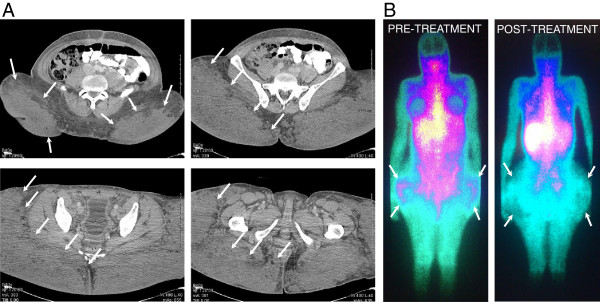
Imaging data showing extensive grannulomatous disease in the hip region. A - Axial computed tomography (CT) images showing large amounts of diffusely infiltrating granulomatous tissue (arrows). B - Gallium-67 uptake scan showing diffuse tracer uptake in the perigluteal soft tissue, corresponding to the subcutaneous densities on physical exam. Images were taken before (i) and after (ii) initiation of systemic corticosteroid therapy, improving the patient’s condition over time.
